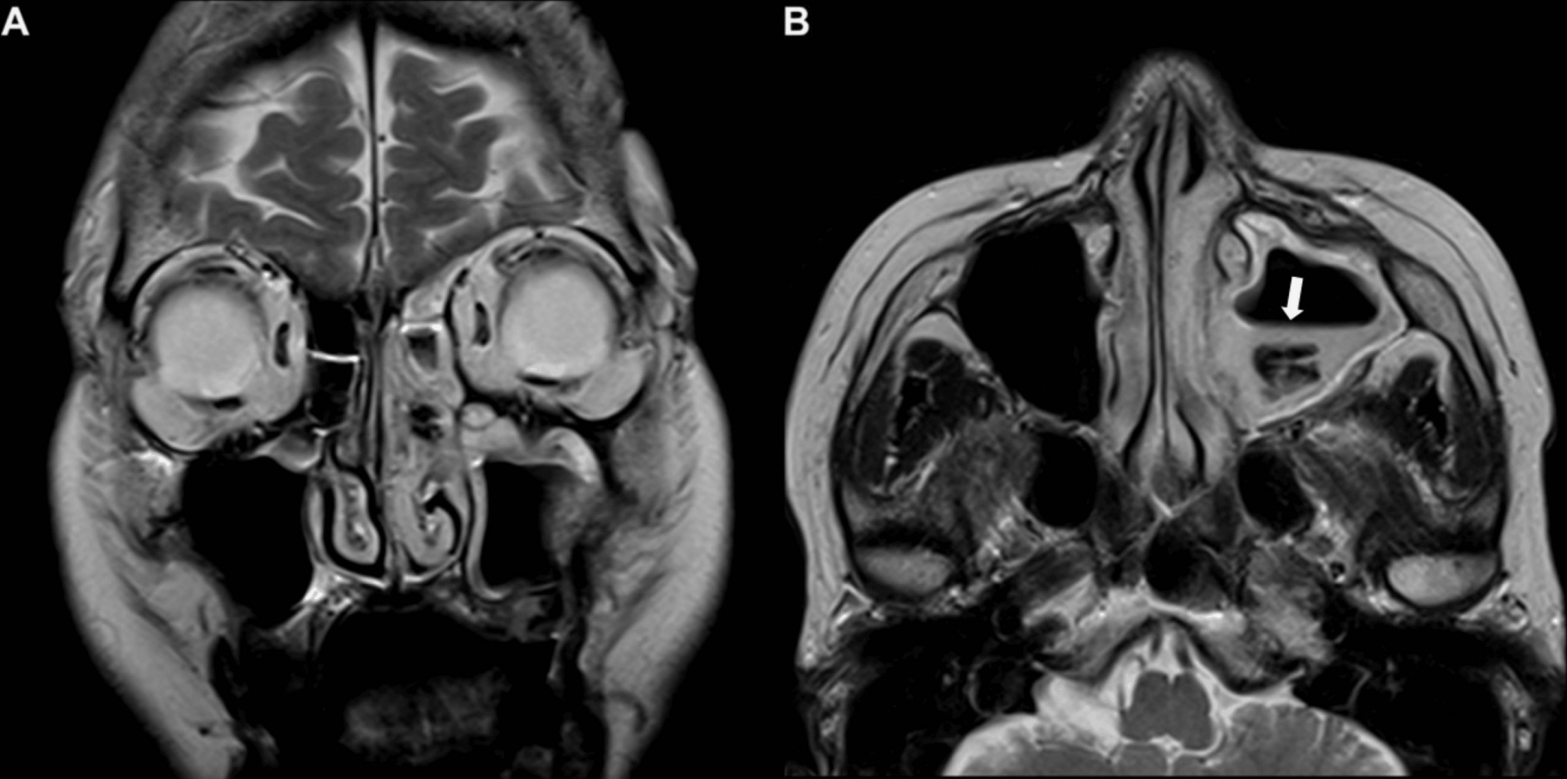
Coronal T2WI (A) showing a high signal intensity along the left maxillary sinus wall, extending to the left ethmoid and frontal sinuses, consistent with mucosal thickening. Axial T2WI (B) showing an air-fluid level (high signal) posteriorly in the maxillary sinus and significant low signal intensity within the sinus corresponding to the high-density area on CT scan (white arrow), which are indicative of a fungus ball.
